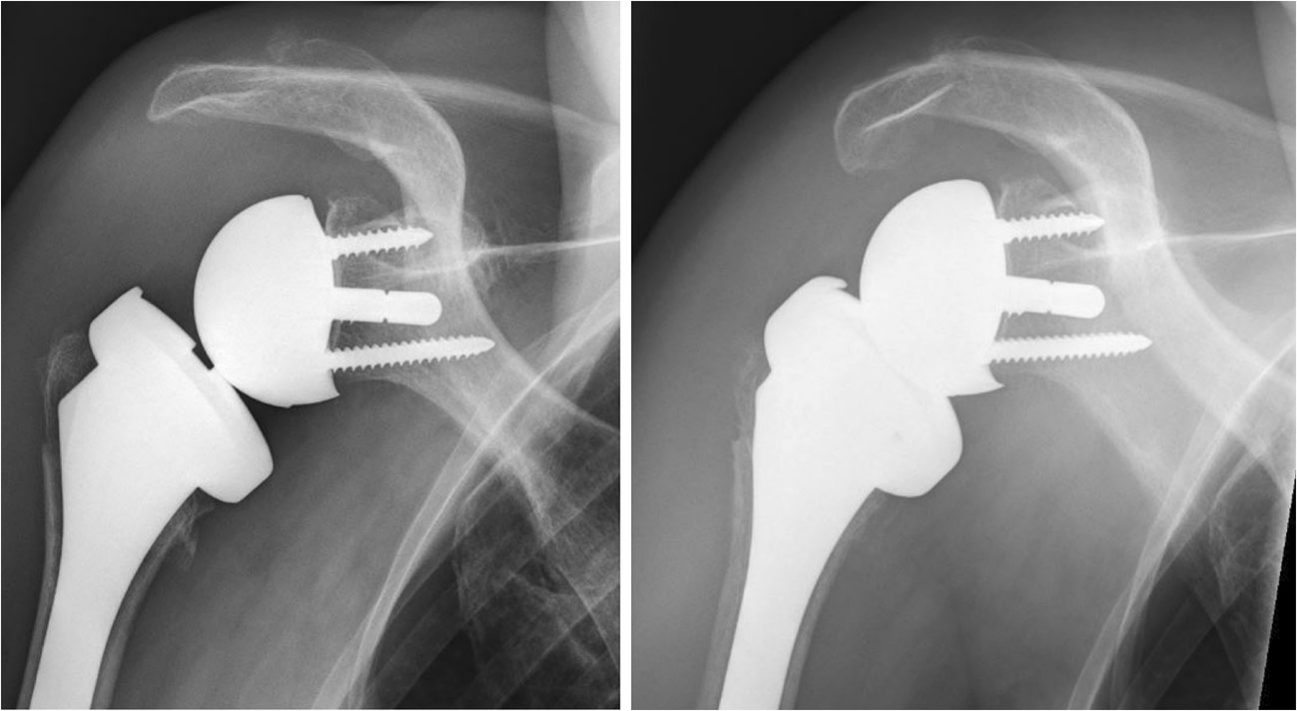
Anteroposterior radiographs of patient 4 treated with a reverse shoulder prosthesis for an acute humeral head fracture after a fall. The patient reported another accidental fall 3 month after surgery. X-ray pictures showed a type I acromion fracture
